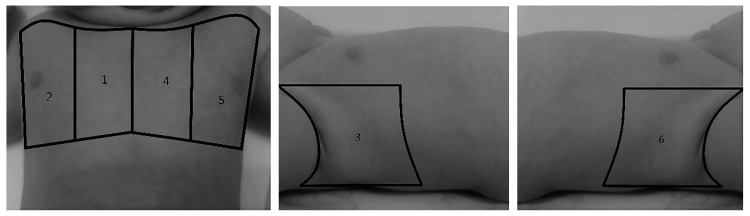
Chest projections used in our study protocol. (1) Right anteromedial (from the parasternal line to right mid-clavicular line), (2) right anterolateral (from the right mid-clavicular line to right anterior axillary line), (3) right lateral (from the right anterior axillary line to right posterior axillary line), (4) left anteromedial (from the parasternal line to left mid-clavicular line), (5) left anterolateral (from the left mid-clavicular line to left anterior axillary line) and (6) left lateral (from the left anterior axillary line to left posterior axillary line).
